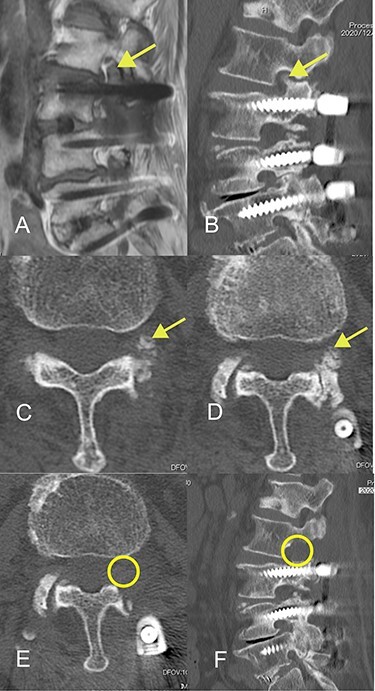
Magnetic resonance and computed tomography (CT) images acquired at the time of onset of radiculopathy. (A) Sagittal T1-weighted magnetic resonance image showing moderate foraminal stenosis at the L2–L3 level. (B) Sagittal CT image showing ossification of the ligamentum flavum protruding into the foramen. (C, D) Axial CT image showing OLF in the capsular portion. (E, F) Postoperative CT image confirming adequate removal of the ossification of the ligamentum flavum.
